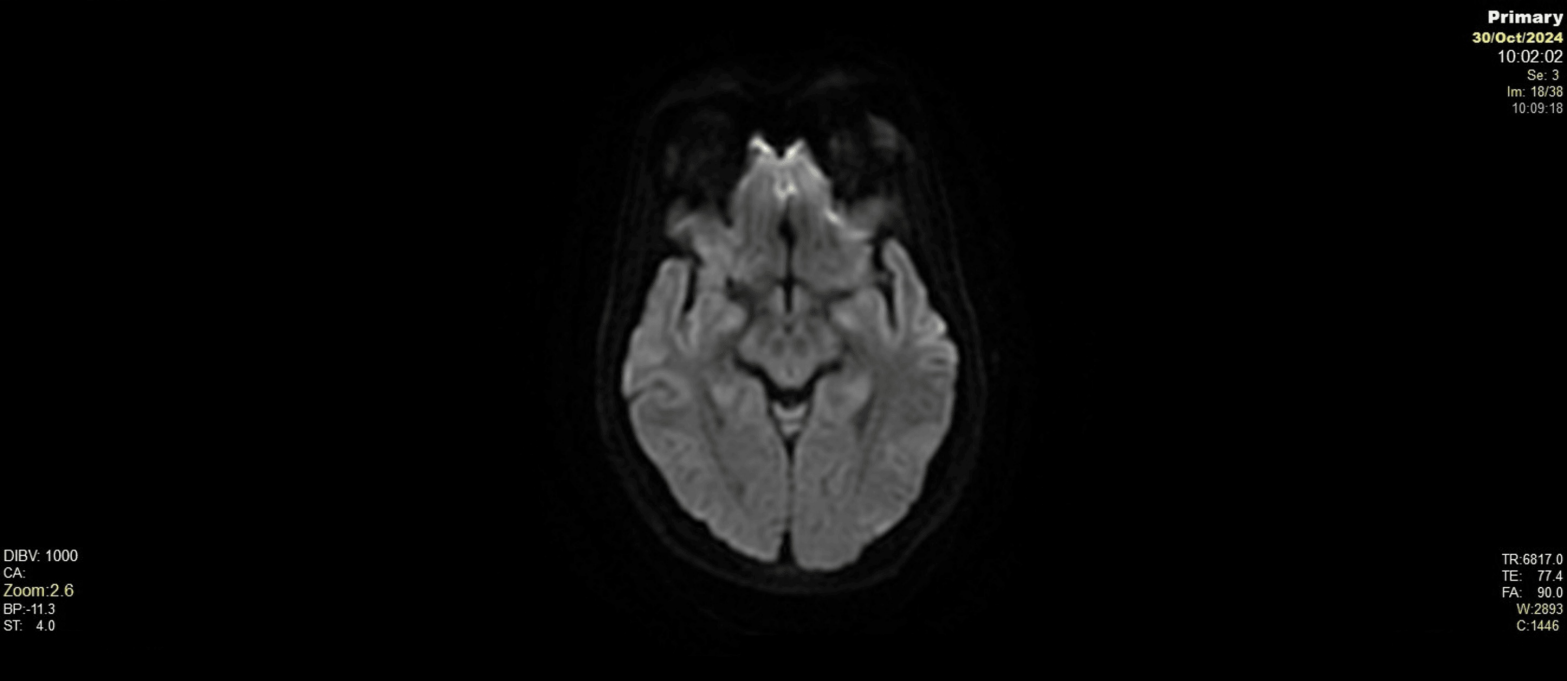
Axial diffusion-weighted imaging (DWI) No areas of restricted diffusion seen on DWI, excluding acute ischemia or cytotoxic oedema. Findings are within normal limits.
